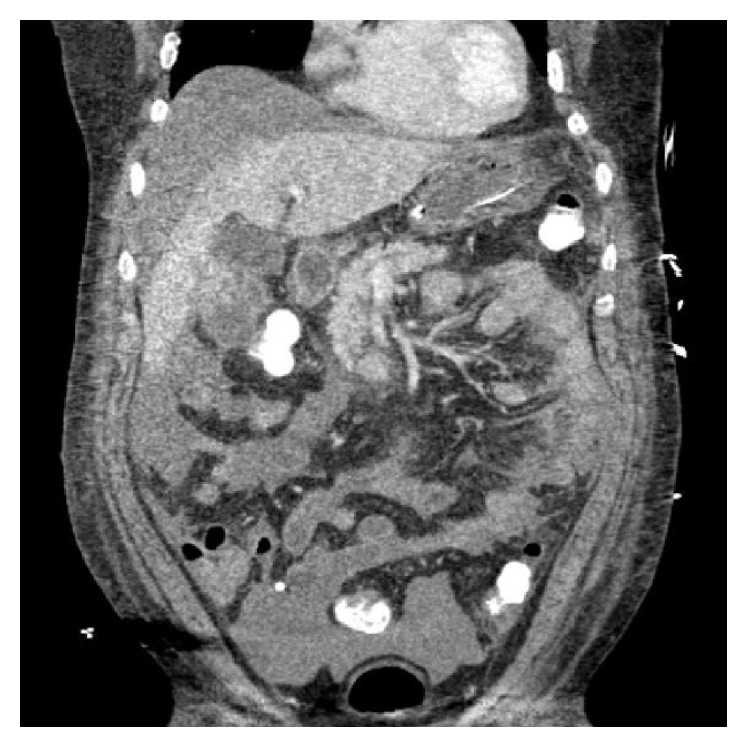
CT scan of abdomen and pelvic showing a mass at segment 5 of liver.
